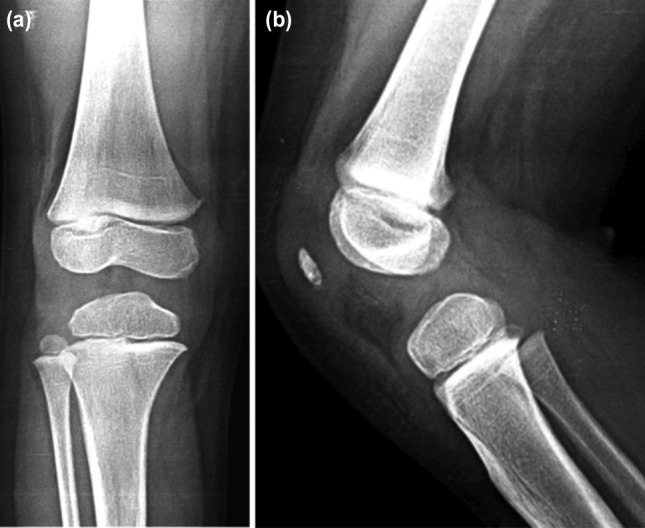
(a) Pre-operative anteroposterior and (b) lateral radiographs of the right knee before femoral derotational osteotomy surgery.
